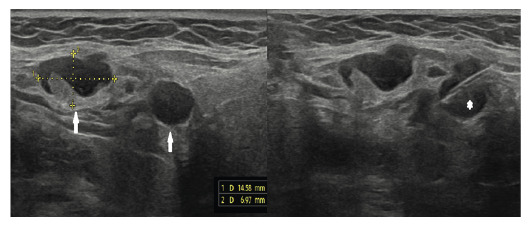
Ultrasound image demonstrating thickened cortex and reactive lymph nodes (white arrows). Fine-needle aspiration biopsy was performed (white star).
